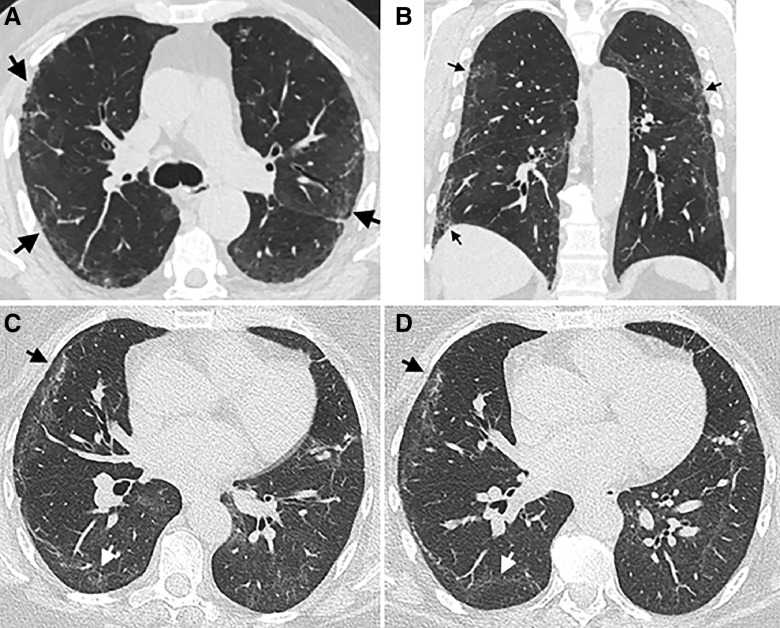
Representative computed tomography (CT) images of residual lung abnormalities. Representative (*A*) coronal and (*B*) axial noncontrast CT imaging from the same individual performed 137 days after discharge after a coronavirus disease (COVID-19) admission scored with 52.5% total lung involvement of residual lung abnormality, of which 18.3% was reticulation and 34.2% ground-glass opacity. Peripheral reticulation (arrows) is evident, surrounded by faint areas of ground-glass opacity. Representative coronal CT images from the same individual at (*C*) 114 days after discharge scored 56.8% lung involvement (28.5% reticulation and 28.3% ground-glass opacity), and (*D*) 239 days after discharge scored 49.2% total lung involvement (20.0% reticulation and 29.2% ground-glass opacity). Peripheral areas of reticulation (black arrow) and ground-glass opacity (white arrow) in the right lung.
